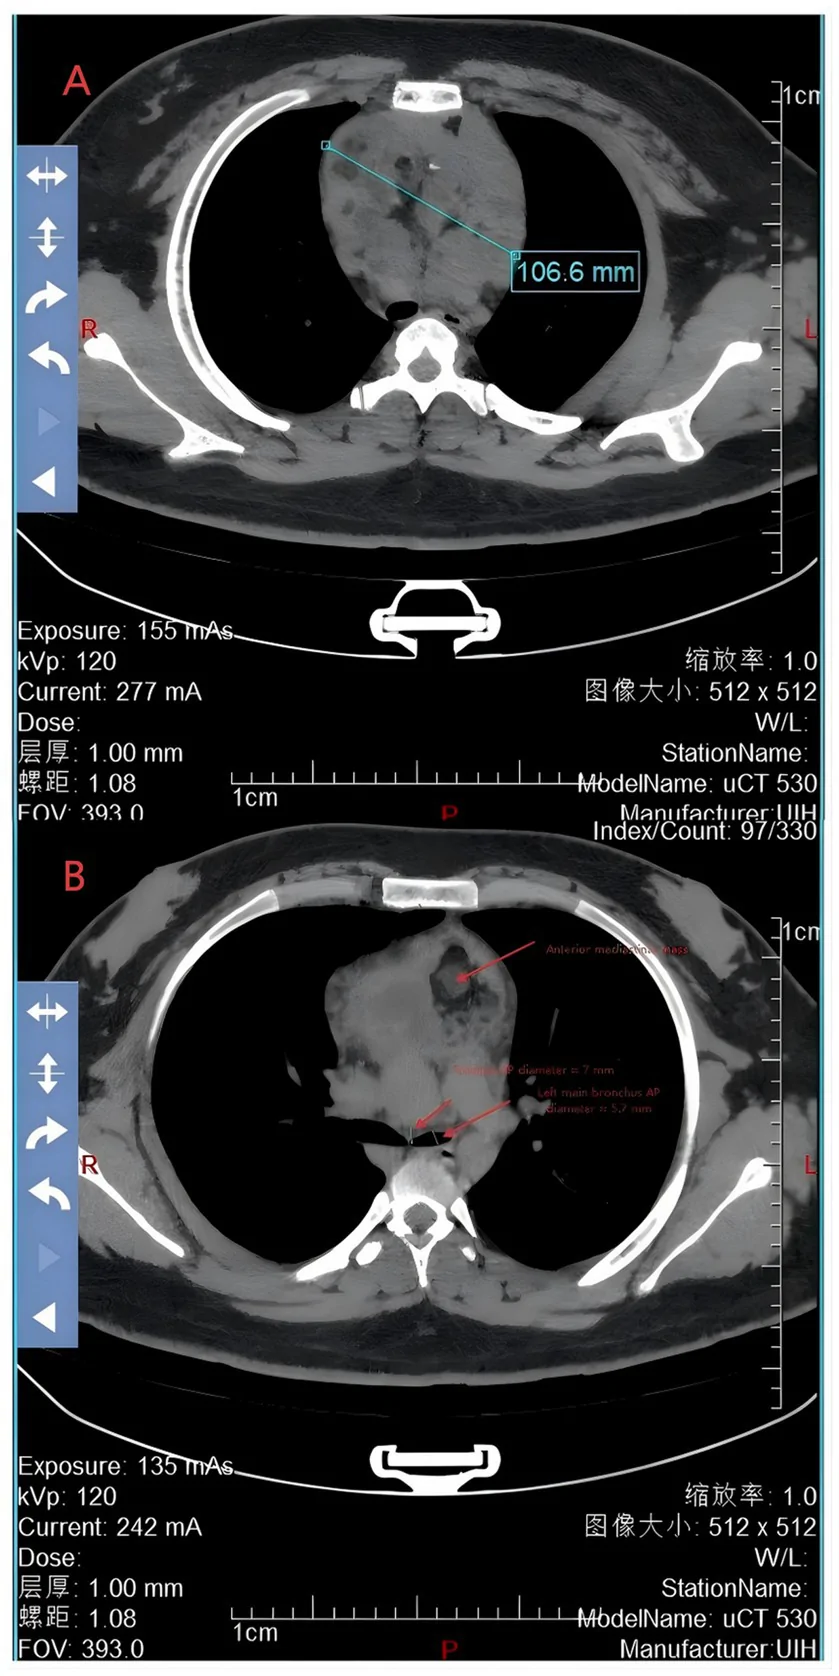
Preoperative contrast-enhanced chest CT (mediastinal window). (A) Axial image at the level of maximum tumor cross-section showing a 10.4 × 9.7 cm heterogeneous anterior mediastinal mass with mixed soft-tissue, fluid, calcified, and fat-density components. The mass displaced the heart to the left. (B) Axial image at the carina level demonstrating posterior compression of the trachea (anteroposterior diameter ≈ 7 mm, ~50% stenosis) and marked narrowing of the left main bronchus (anteroposterior lumen ≈ 5.7 mm, ~55% stenosis). The carina is deformed and displaced.
